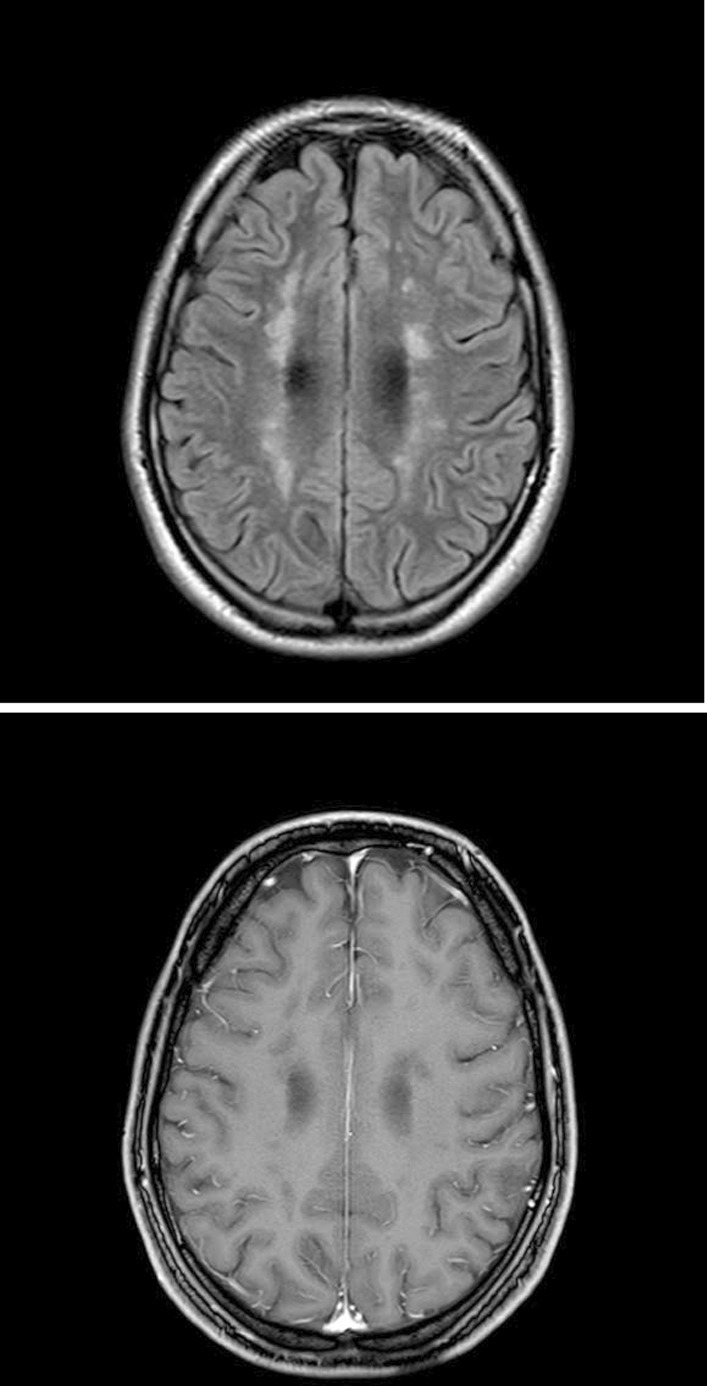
Follow-up 3 months after initial presentation: The FLAIR sequence depicts a reduction in size of the white matter lesions without pathological/corresponding contrast enhancement
